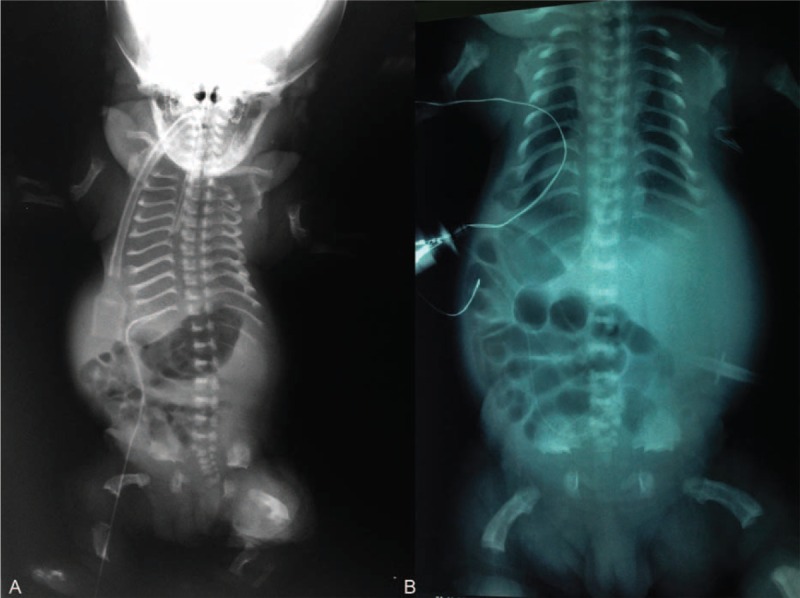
Radiographs of case 2 (A) and case 3 (B) show small thorax, and short and horizontal ribs with cupped anterior ends. The calvarium is relatively large (A). The humeri are curved and short. Platyspondyly with an H appearance of the vertebrae is noted. The ilia are small and squared, with a horizontal, trident-shaped acetabular roof. The greater sciatic notches are very small. The ischia and pubis are small, with widening of the symphysis and the sacroiliac joints. The femora show a “telephone receiver” shape. The femora are widened proximally with an oval area of decreased opacity similar to that seen in newborns with achondroplasia.
